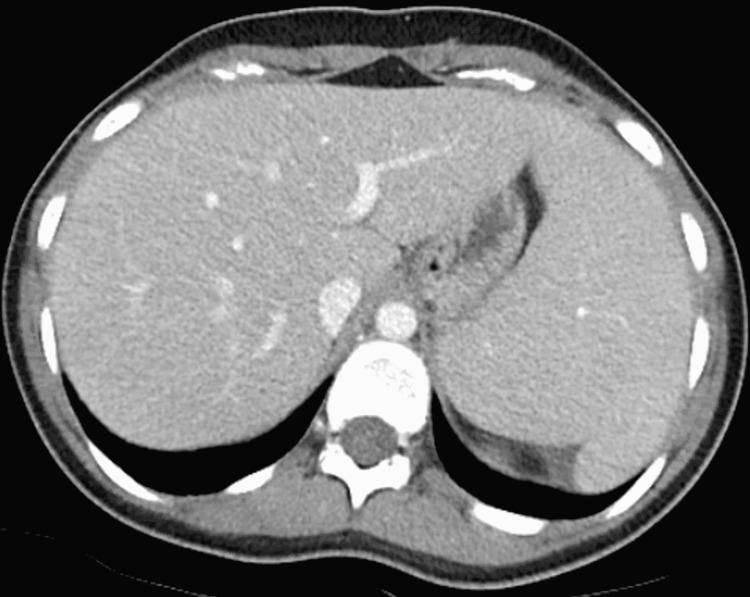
Computed tomography of the abdomen revealing splenomegaly but no other relevant findings.
